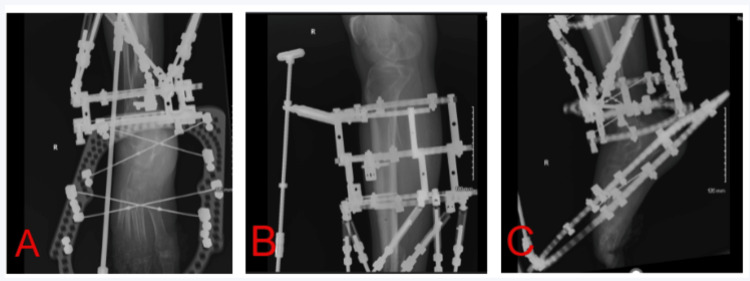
Direct postoperative X-ray imaging. A: Anteroposterior radiographic imaging of the distal right tibia and foot showing existing Taylor spatial frame, dual fine wire placements through the calcaneus and metatarsals, and a foot plate with a pulling rod. B: Lateral radiographic imaging of the right proximal tibia showing existing Taylor spatial frame with a rancho cube hinge construct connecting the frame to the pulling rod. C: Lateral radiographic imaging of the right distal tibia and foot following foot plate attachment to the existing Taylor spatial frame. Note the absence of any hardware connection between the foot plate and distal tibial Taylor spatial frame. The ankle is used as a natural hinge to facilitate dorsiflexion.
